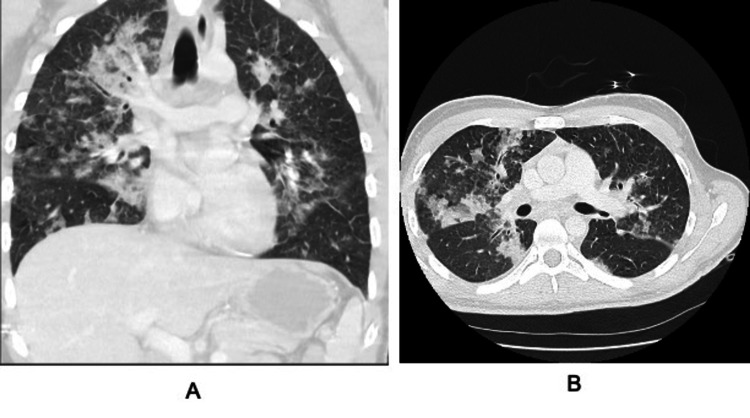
CT scans showing diffuse bilateral infiltrates with ground glass opacities and no evidence of pulmonary embolism.
